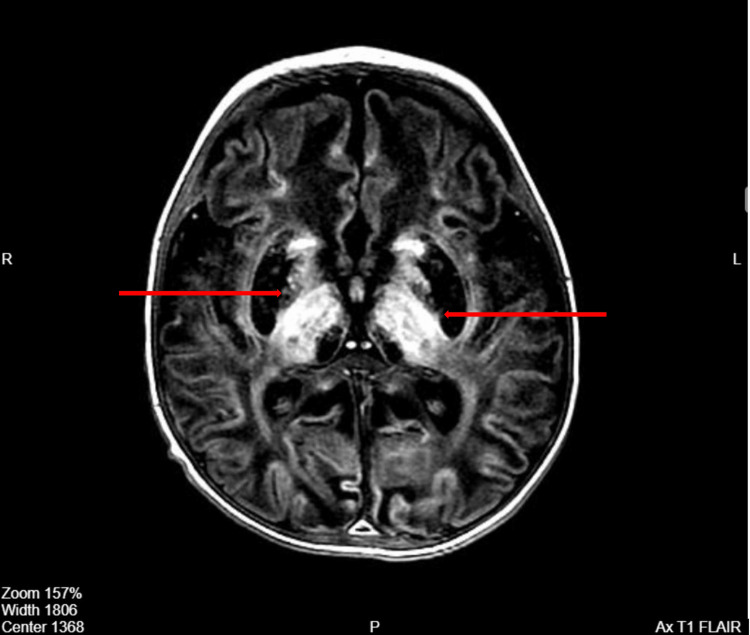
Brain MRI T1 FLAIR sequence showing abnormal signal intensity on the basal ganglia and thalami on both sides FLAIR: Fluid-attenuated inversion recovery
